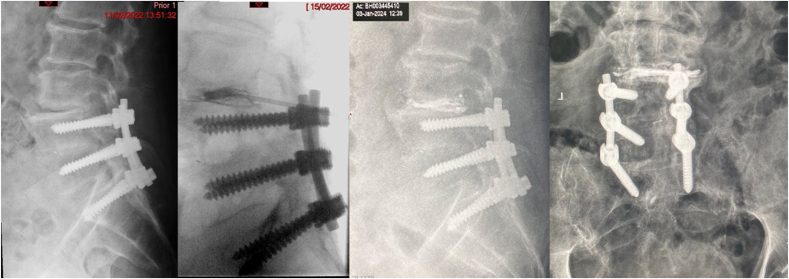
84 year old man with back pain 7/10 and leg pain 5/10, with kidney transplant, early dementia and low BMI, walking-frame reliant, having received L4L5S1 fusion over 15 years previously. Had percutaneous CD. A: Pre-operative radiograph. B: Intra-operative fluoroscopy with trocar in situ, Kambin's triangle approach, at L34 mid-cementation, small cement leak (not in spinal canal or foramena). C & D: Two-year post-operative radiographs. No peri-operative or early complications. VAS had improved to 2/10 in his back, no leg pain, walking stick reliant. ODI had improved from 72% to 28%.
